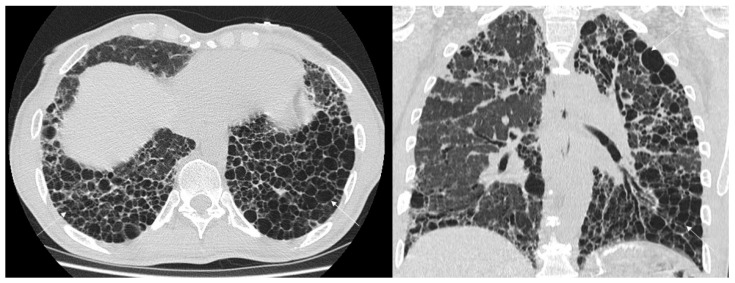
High-resolution computed tomography (HRCT) of the chest in axial (left) and coronal (right) views showing exuberant honeycombing, characterized by extensive, confluent subpleural cystic airspaces with well-defined walls and similar diameters, predominantly in the lower lobes and posterior regions.
